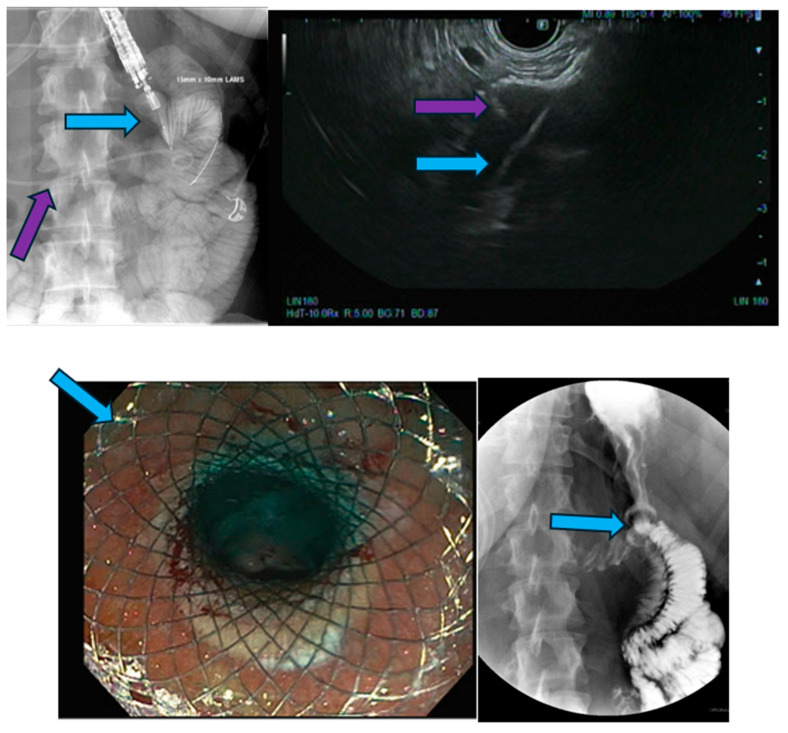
Fluoroscopy image [upper left] reveals a distal stent delivery of a 15 mm × 10 mm lumen-apposing metal stent (blue arrow) from the stomach to the small bowel. A nasobiliary drain (purple arrow) was used to instill contrast and methylene blue to localize and distend the small bowel. Endoscopic ultrasound image [upper right] reveals needle puncture (blue arrow) from the gastric wall to the small bowel. Note the delivery of the distal flange of the LAMS (purple arrow). Endoscopic image [lower left] of a fully deployed LAMS (blue arrow). Upper gastrointestinal series with oral contrast [lower right] demonstrates passage of oral contrast into the small bowel, which demonstrates stent patency (blue arrow).
